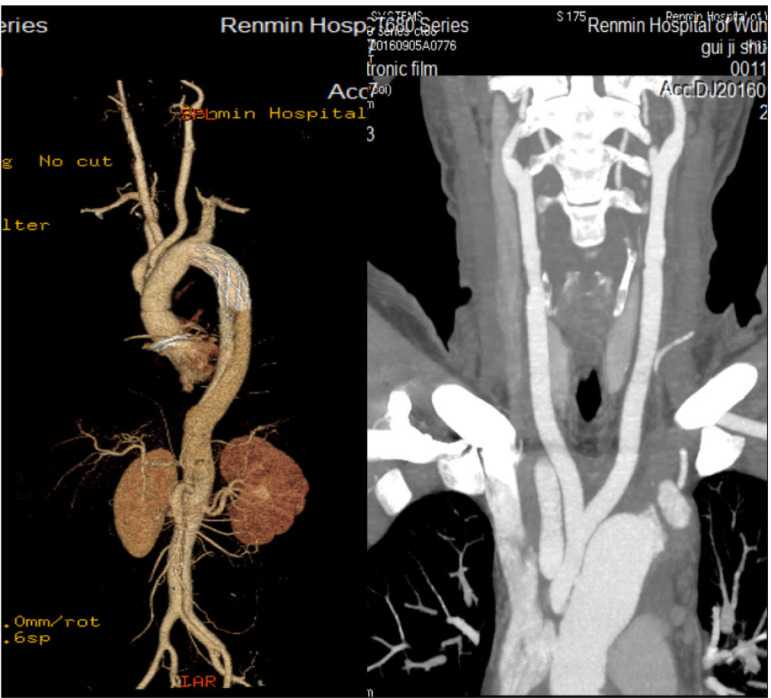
This postoperative computed tomography angiography of Case 1 demonstrates that the artificial vessels of bilateral common carotid arteries had patency without dissection.
